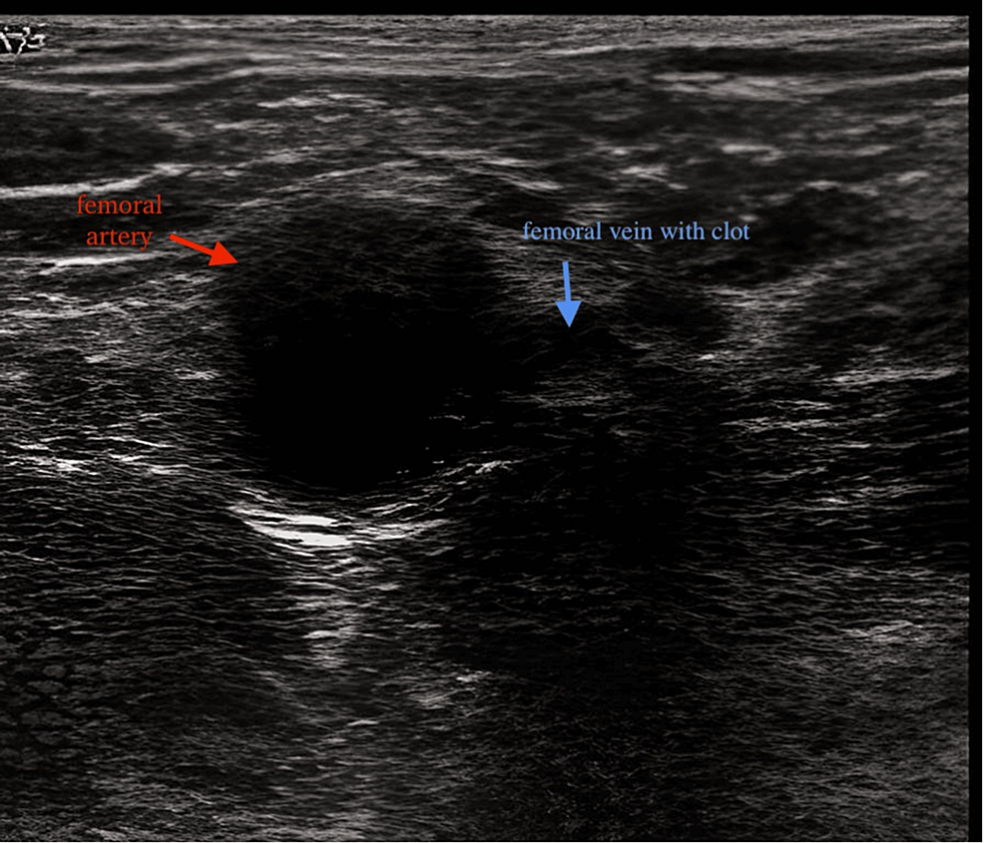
Thrombosis of the right femoral vein on Doppler ultrasound.
